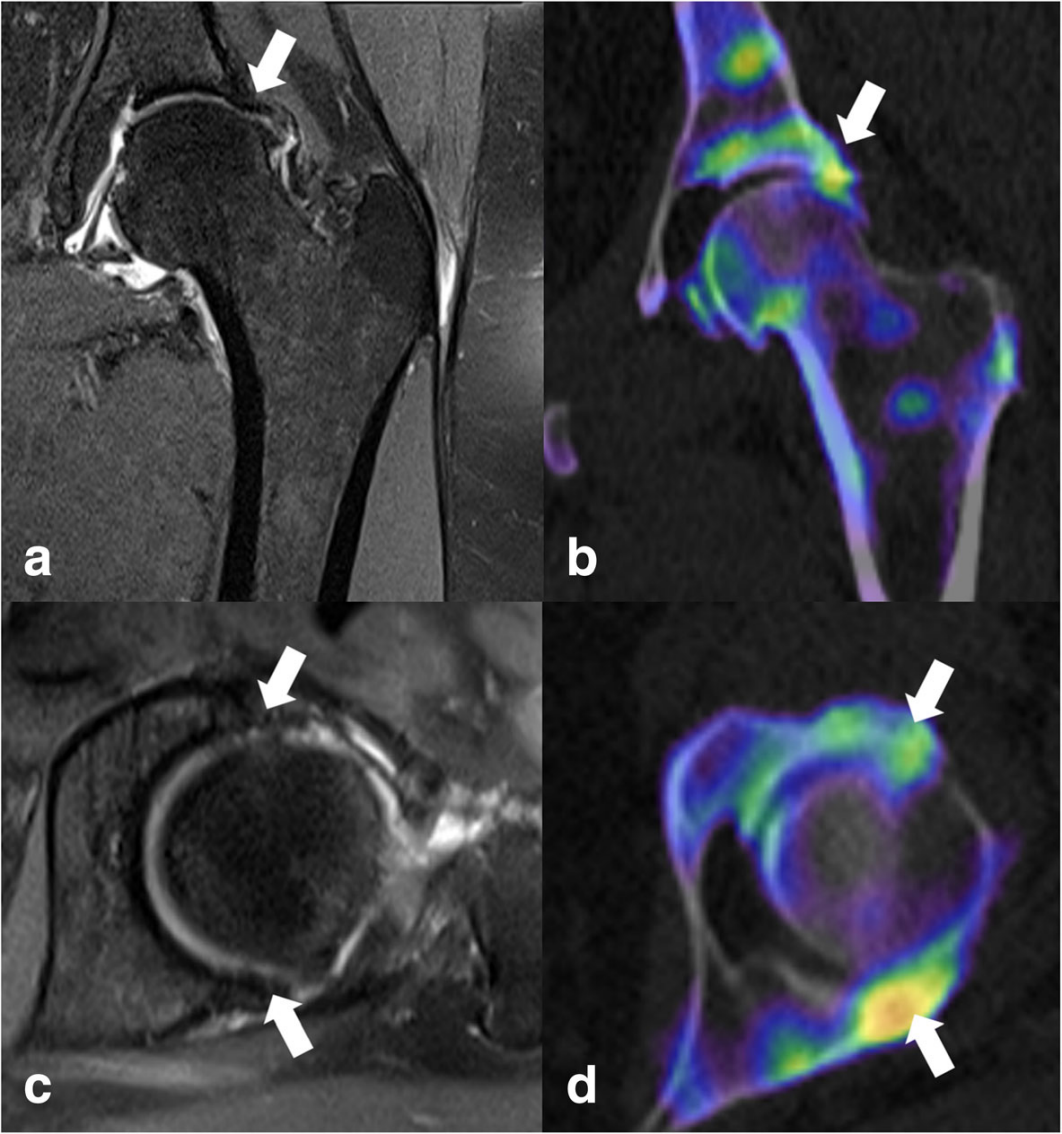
MRI and xSPECT/CT of an osteoarthritic left hip. Detailed Legend: Corresponding slices from the same patient: a Coronal T2 fat suppressed image, b Coronal xSPECT-CT image, c Axial T2 fat suppressed image and d Axial xSPECT-CT images in the same patient. Thick arrows show cartilage loss (MRI) with corresponding adjacent bone changes (xSPECT/CT)
